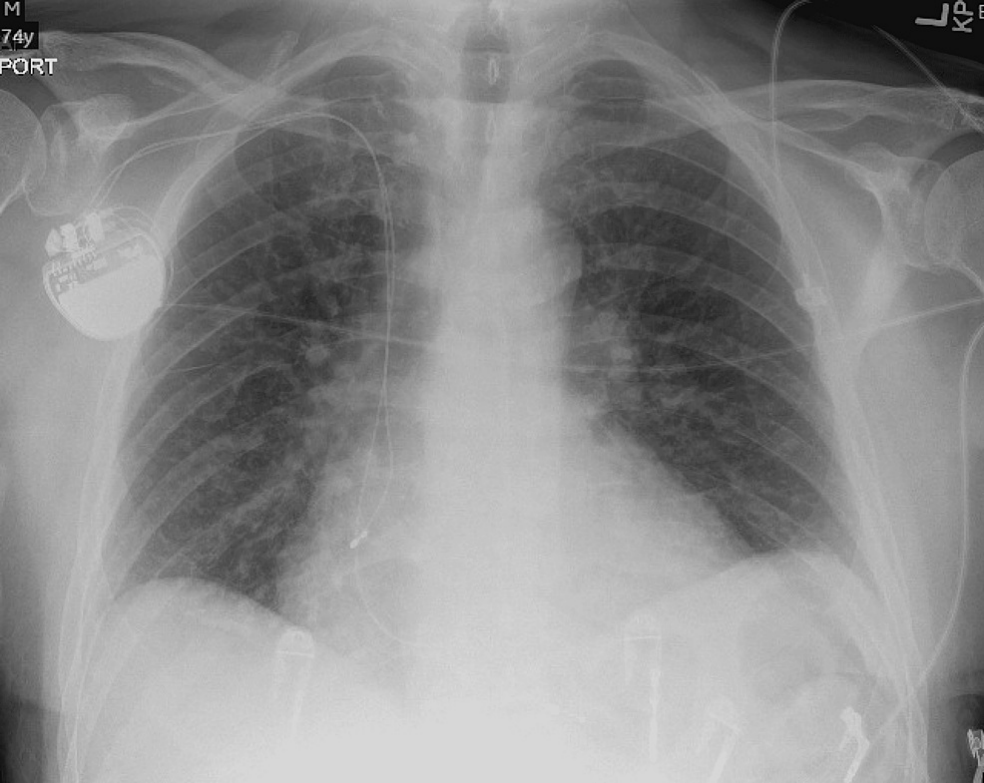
Chest X-ray showing dual-chamber pacemaker with intact leads
